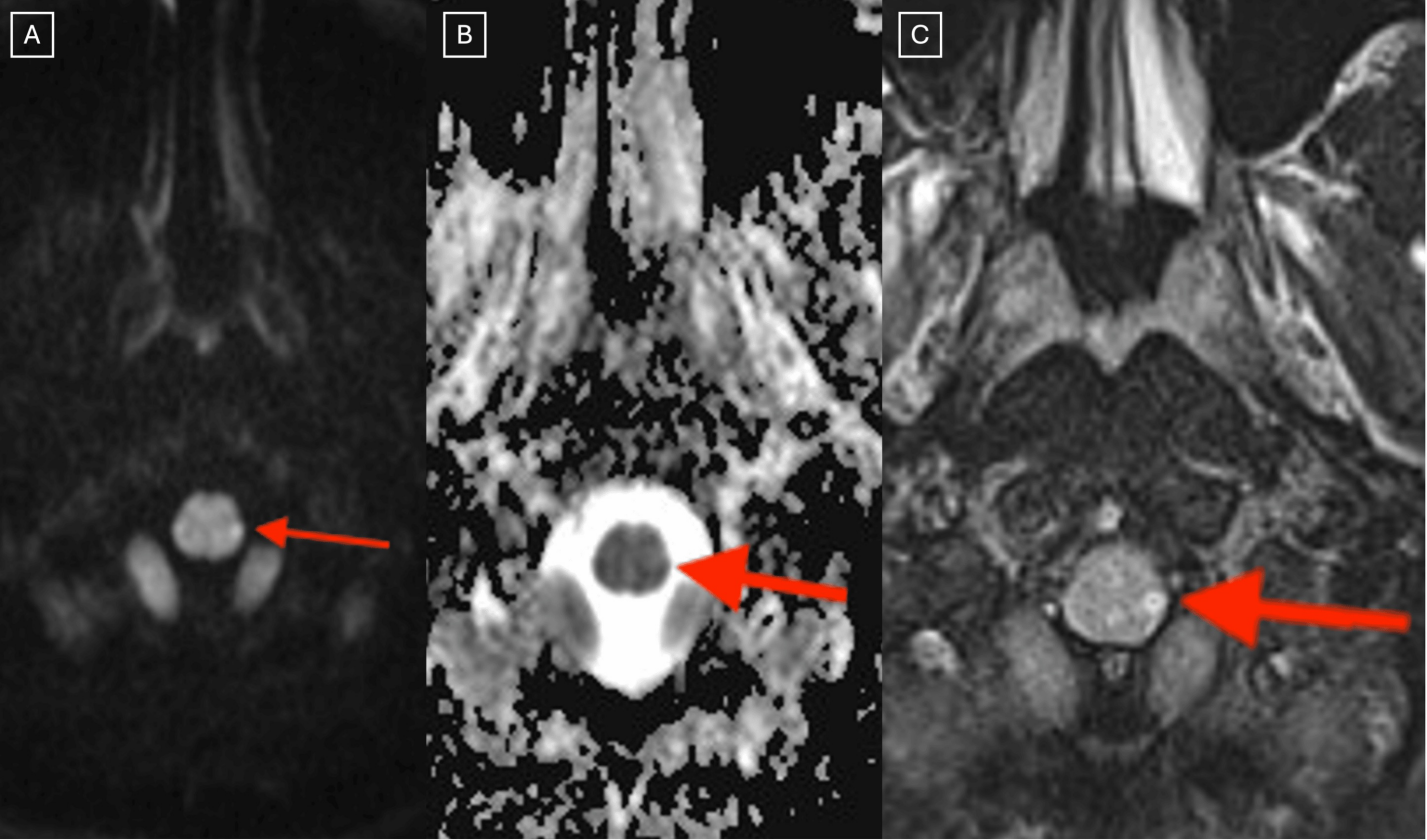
Side-by-side comparison of transverse diffusion-weighted (A), apparent diffusion coefficient (B), and T2-FLAIR (C) MRI of the brain at the level of the pyramidal decussation. The infarct location is indicated by a red arrow. FLAIR: fluid-attenuated inversion recovery
